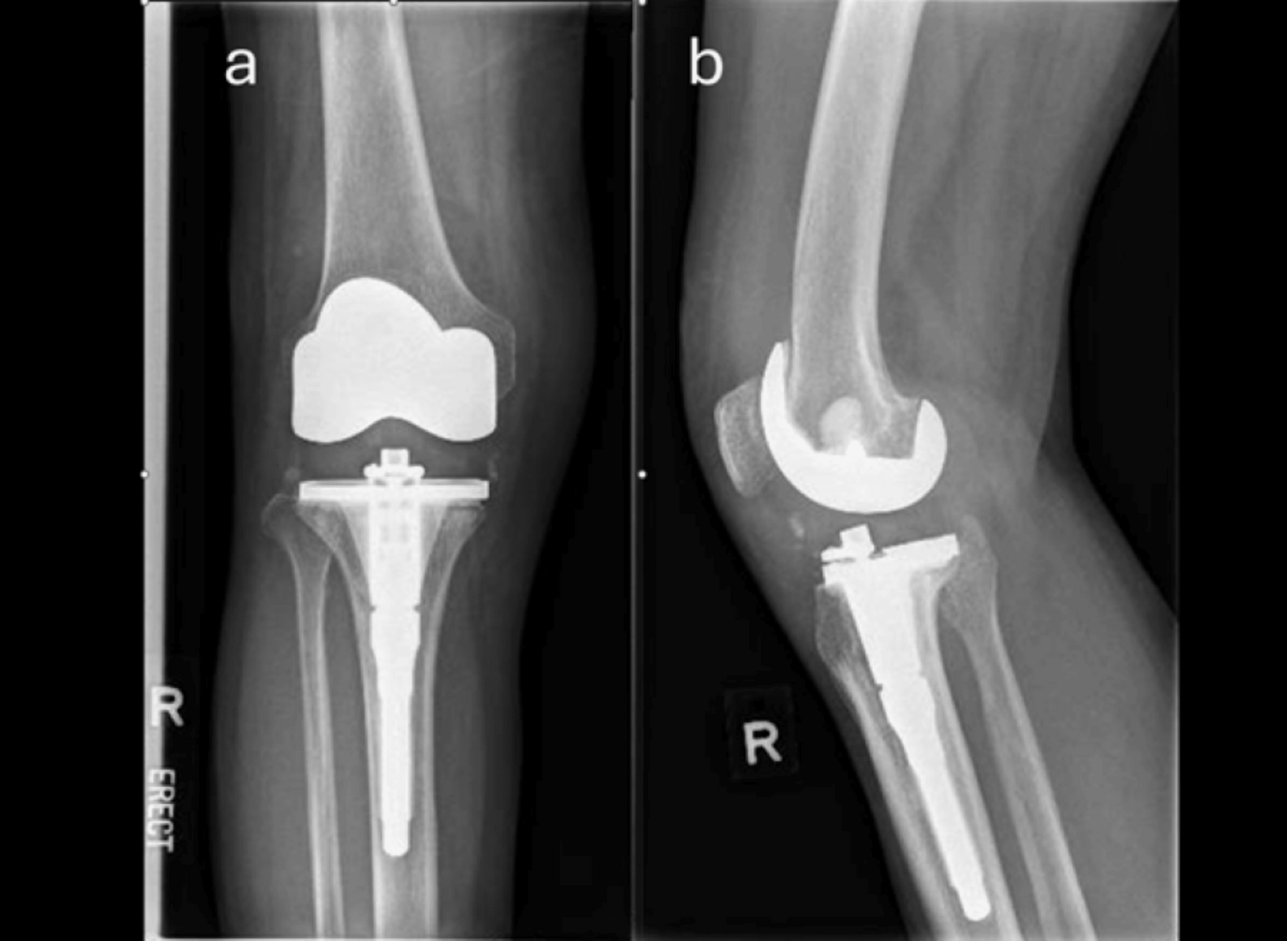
Postoperative knee radiograph. (a) Antero-posterior view; (b) Lateral view
